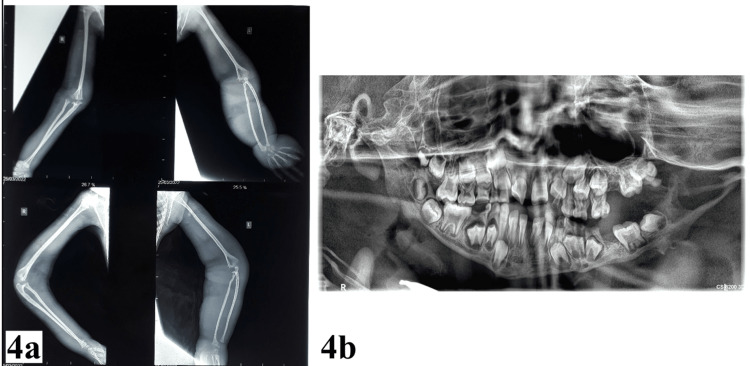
Plain radiographs. (a) X-ray of upper limbs showing dysplastic long bones and left macrodactyly; (b) there is agenesis of 38 and the morphology of the 34 is suggestive of germination.
